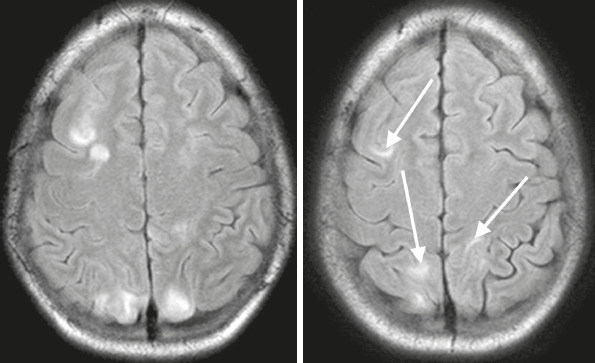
Follow-up brain MRI. Axial T2-weighted FLAIR sequence showing lesions that did
